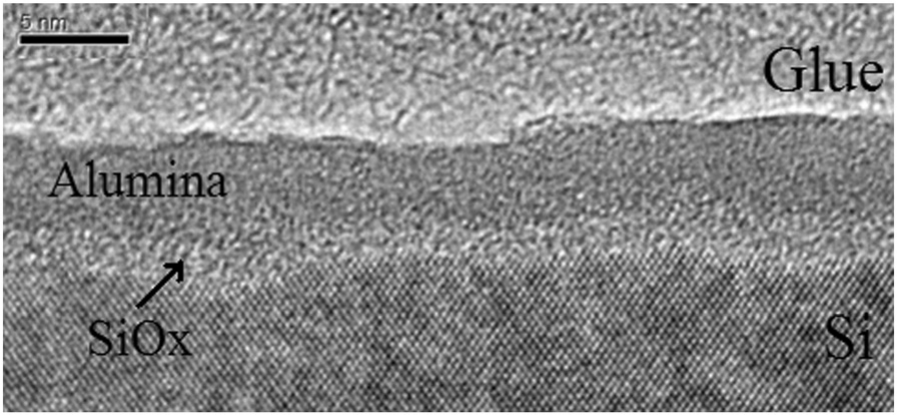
Doppler broadening spectroscopy of *S*-*W *parameters vs. positron incident energy. (a)*S *and (b)*W *parameters vs. positron incident energy for samples annealed at different temperatures for 10 min. (c)*S*-*W* plot for samples annealed at different temperatures for 10 min.
